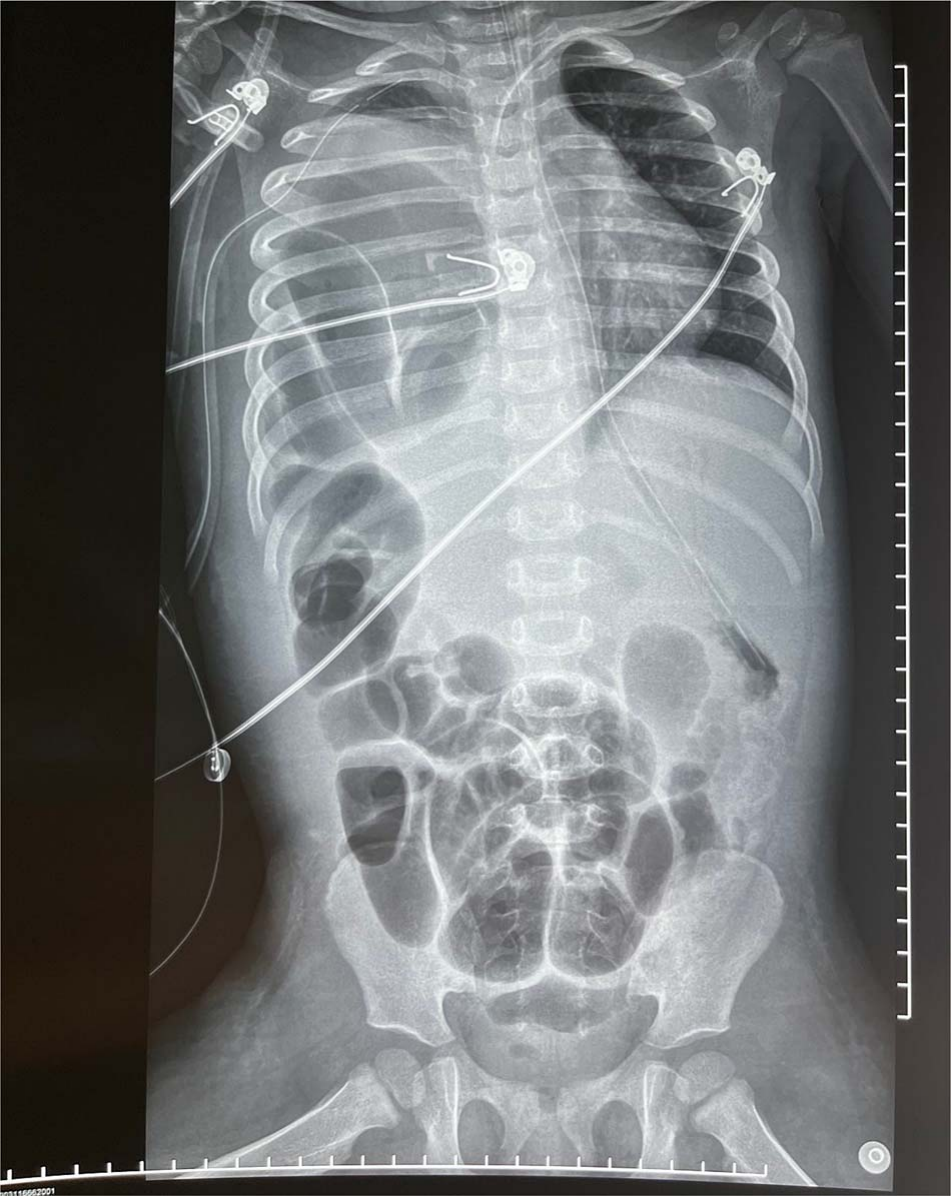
Anteroposterior X-ray showing intestinal loops in the right hemithorax with associated contralateral displacement of the mediastinal structures.
